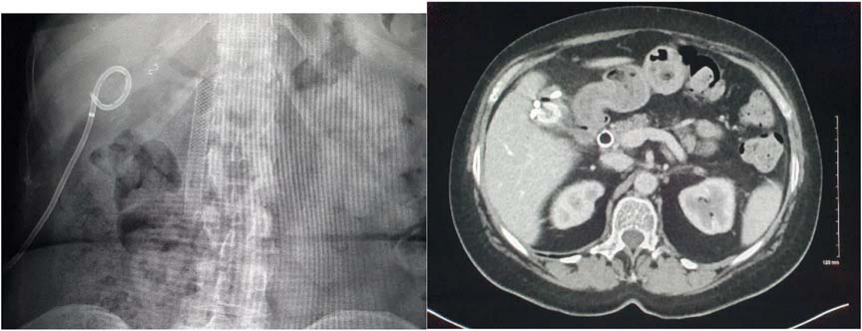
Radiographic and CT images demonstrating the patients’ hardware at discharge with an endoscopic biliary stent and gallbladder fossa pigtail drain *in situ*.
